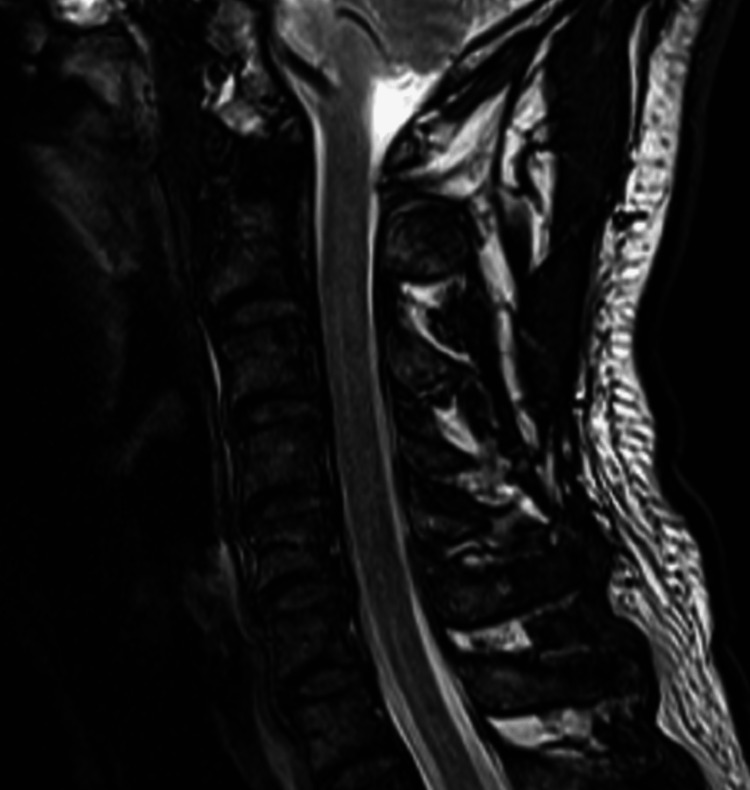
Resolution of the cervical epidural hematoma This mid-sagittal, T2-weighted turbo spin-echo magnetic resonance image taken at the end of the 10th week of the patient’s hospitalization reveals no sign of the previously identified hematoma. Other views (not shown) likewise did not demonstrate any residual hematoma.
